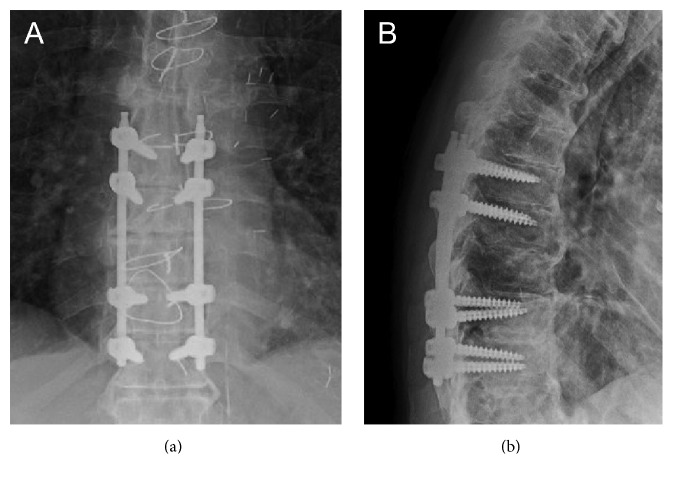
Postoperative thoracic spine X-rays ((a) anteroposterior; (b) lateral) at 3-month follow-up visit showing T7–T11 posterior fixation.
